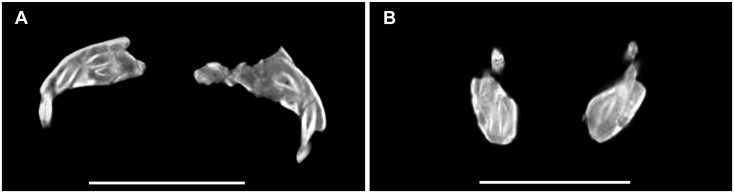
Tooth replacement in *Sarmientosaurus musacchioi* gen. et sp. nov. (MDT-PV 2) as revealed by computed tomography. (A) Transverse section through maxillae showing three replacement teeth in the right maxilla (on the left side of the image) and two in the left. (B) Transverse section through dentaries showing replacement teeth. Scale bars = 10 cm.
